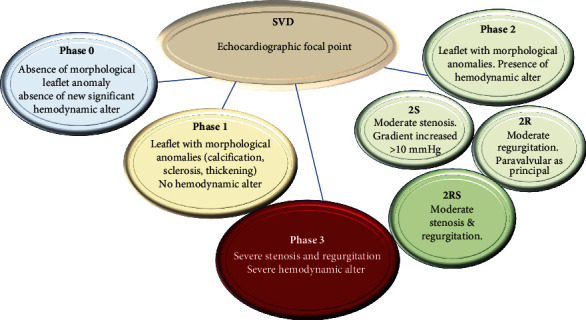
Echocardiographic focal point of the SVD of the stent/stentless xenograft. Infective endocarditis, valve thrombosis, and isolated patient-prosthesis mismatch without functional valve deterioration, isolated paravalvular regurgitation, and frame distortion without abnormal leaflet function are excluded at this focal point of SVD. However, these conditions may explain phase 1 SVD because these bioprostheses could be inclined to early SVD.
